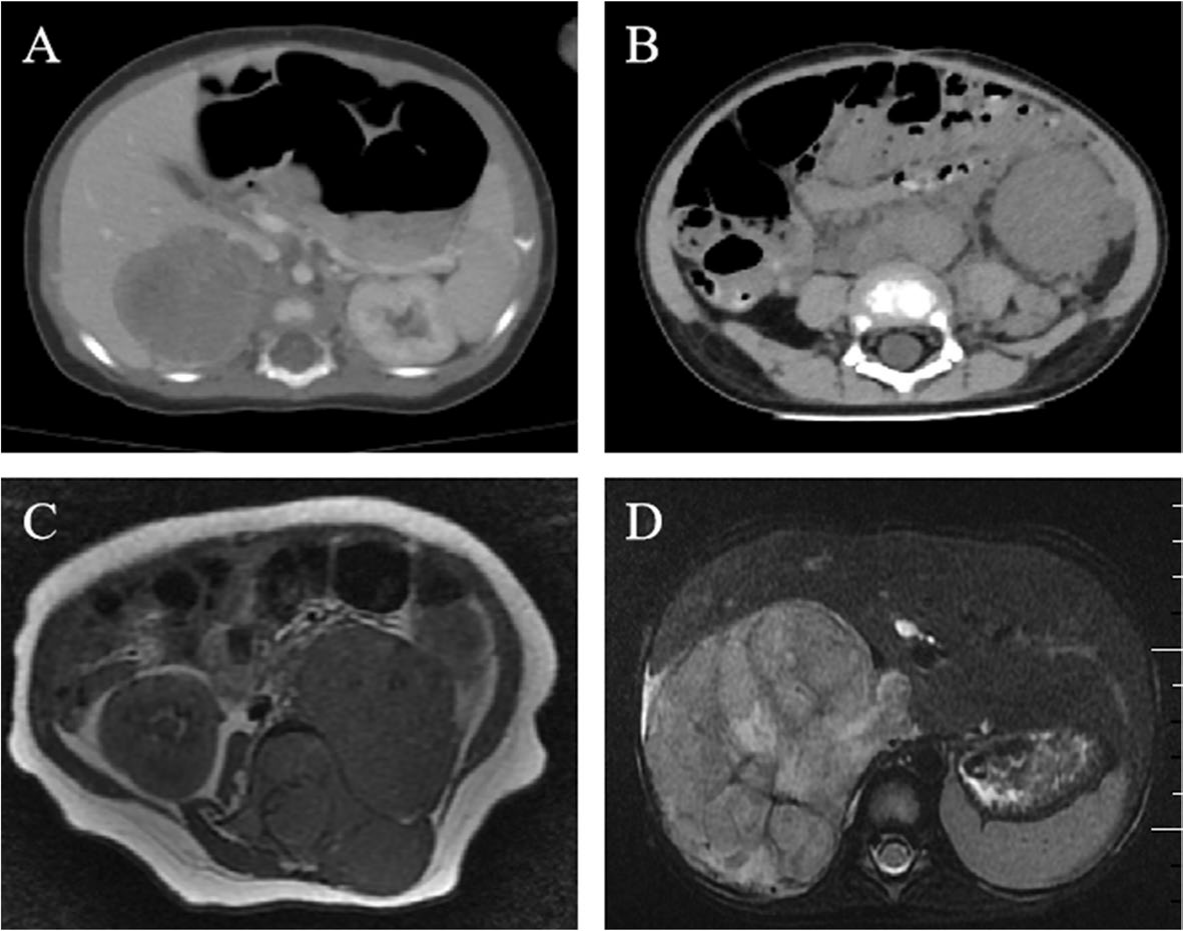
Imaging features. A CT image, male, 2 months, stage I, left adrenal neuroblastoma. The tumor density was not uniform, and irregular low-density areas were inside the tumor. B CT image, female, 15 months, stage III. An irregular mixed density mass with internal gritty calcification and marginal underfinish was observed retroperitoneally. C MRI image, female, 6 months, stage IV. A large substantial mass was behind the left peritoneum. D MRI image, female, 24 months, stage III, right adrenal neurocytoma. Plain scan TWI showed small lamellar vertebrae with slightly elevated signal transfer
